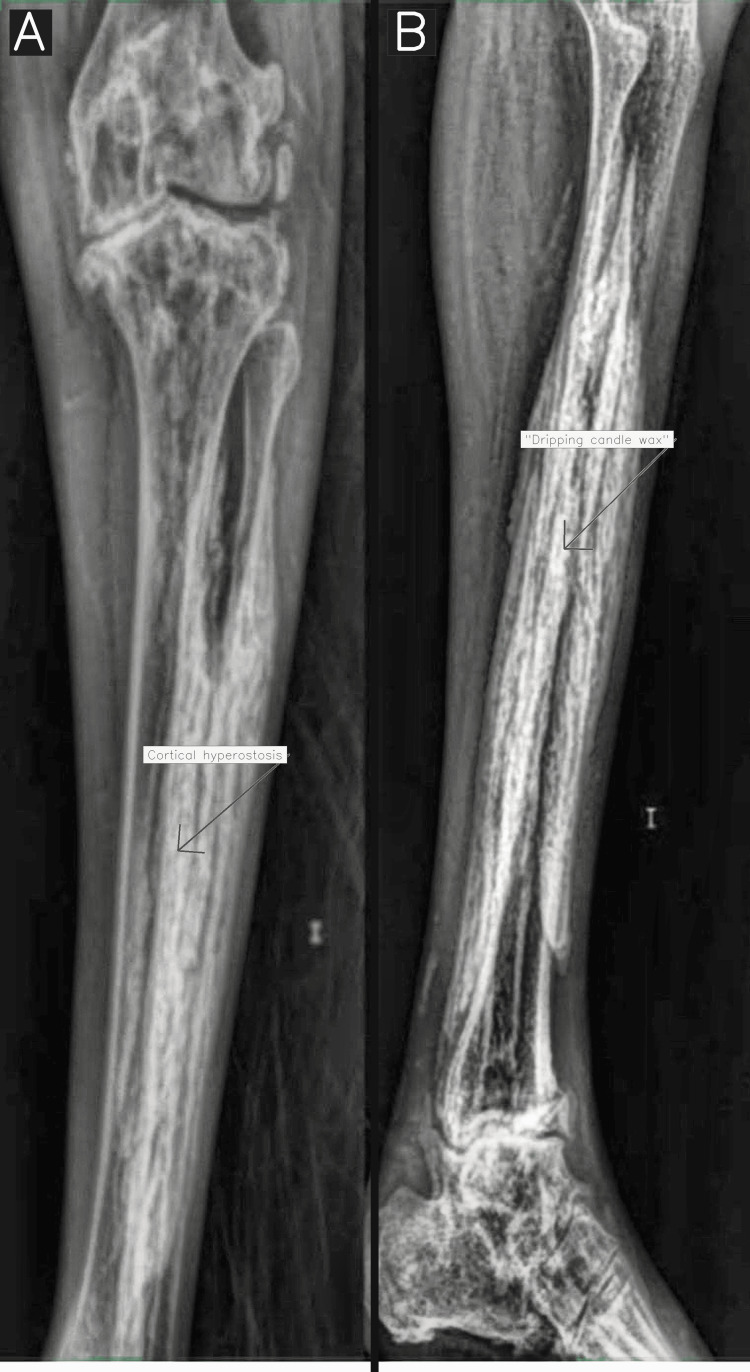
Plain radiographs of the left leg demonstrating melorheostotic hyperostosis. (A) Anteroposterior view showing eccentric periosteal and endosteal cortical thickening of the tibia and fibula with the characteristic “dripping candle wax” appearance. (B) Lateral view confirming the irregular longitudinal hyperostosis along the cortical surfaces.
